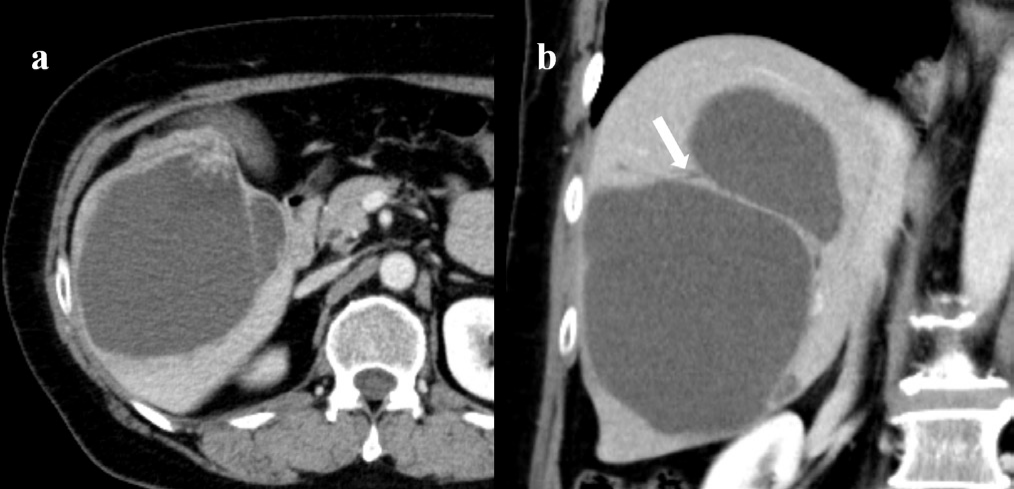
Abdominal contrast-enhanced computed tomography (venous phase). (a) Axial image shows multicystic mass with a solid enhancing mural nodule in the right lobe of the liver. (b) Coronal image shows the mass connecting with B7 (arrow) with slightly upstream dilatation
